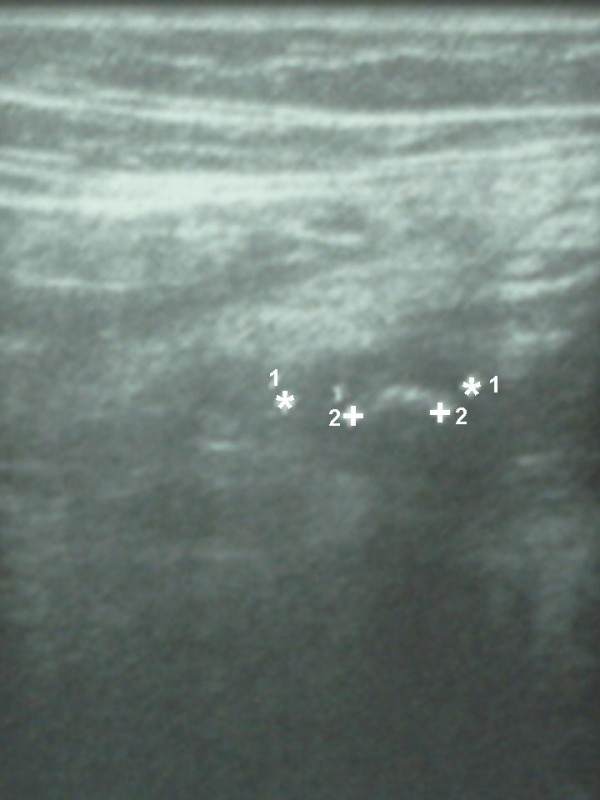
Ultrasonography revealing fecalith (1) into appendiceal stump (2).
